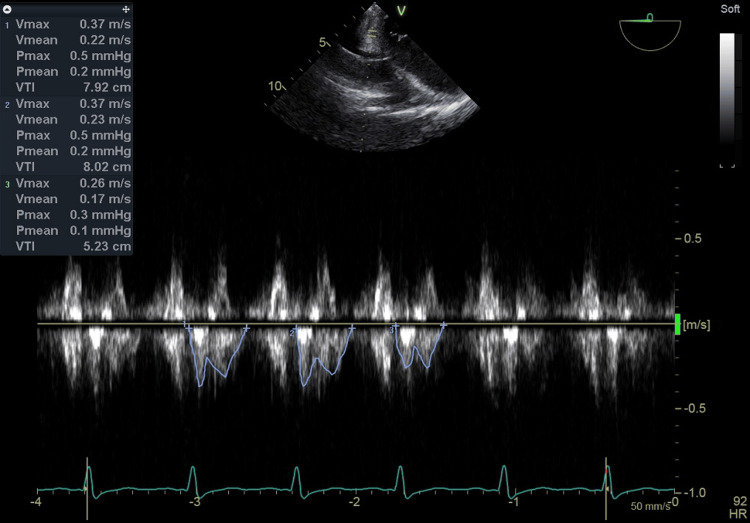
The VTI of CS blood flow measured by the TEE pulse Doppler after induction of anesthesia The VTI value after induction of anesthesia was 7.06 cm (HR 92 beats/min) calculated as the average of three heartbeats excluding premature contractions. In addition, the TEE setting was set to Frames per second 9, Frequency 3.1 MHz, Power 0 dB, Gain -2 dB, Velocity scale 2.0 m/s, Sample volume 4.0 mm, Sample volume depth 2.5 cm and Sweep speed 50 mm/s. VTI: velocity time integral, CS: coronary sinus, TEE: transesophageal echocardiography
